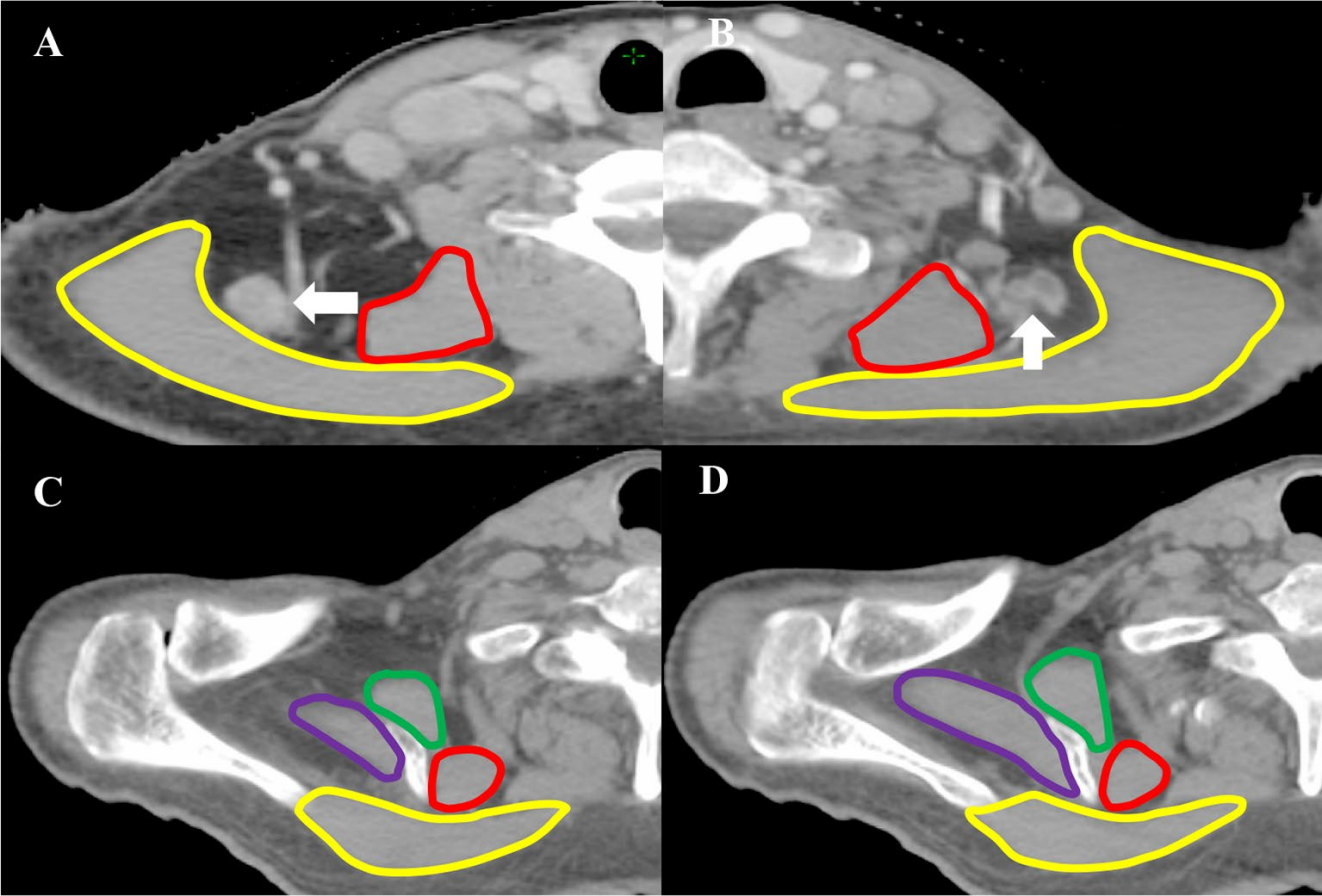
The yellow line represents trapezius, the red line represents levator scapulae, the green line presents the serratus anterior muscle, and the purple line presents the supraspinatus. (A, B) The PLV region in the CT scans, which is between levator scapulae and trapezius. The white arrows indicate the metastatic lymph nodes. (C, D) There is no space between levator scapulae and trapezius when the serratus anterior muscle appears on the CT image.
